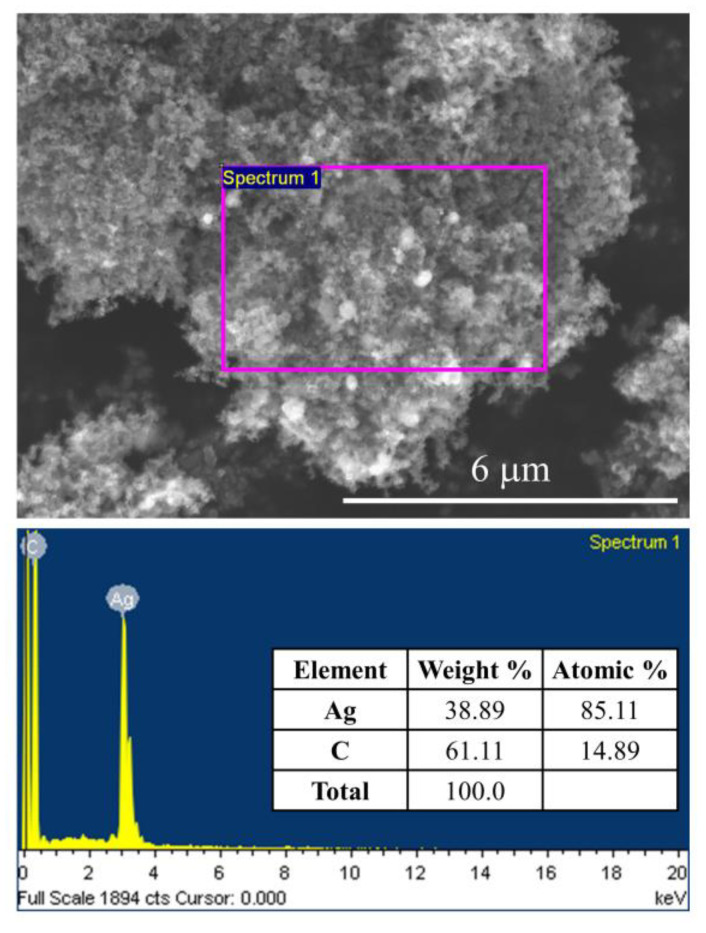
Energy-dispersive X-ray (EDX) pattern of typical Ag/CB catalyst.
